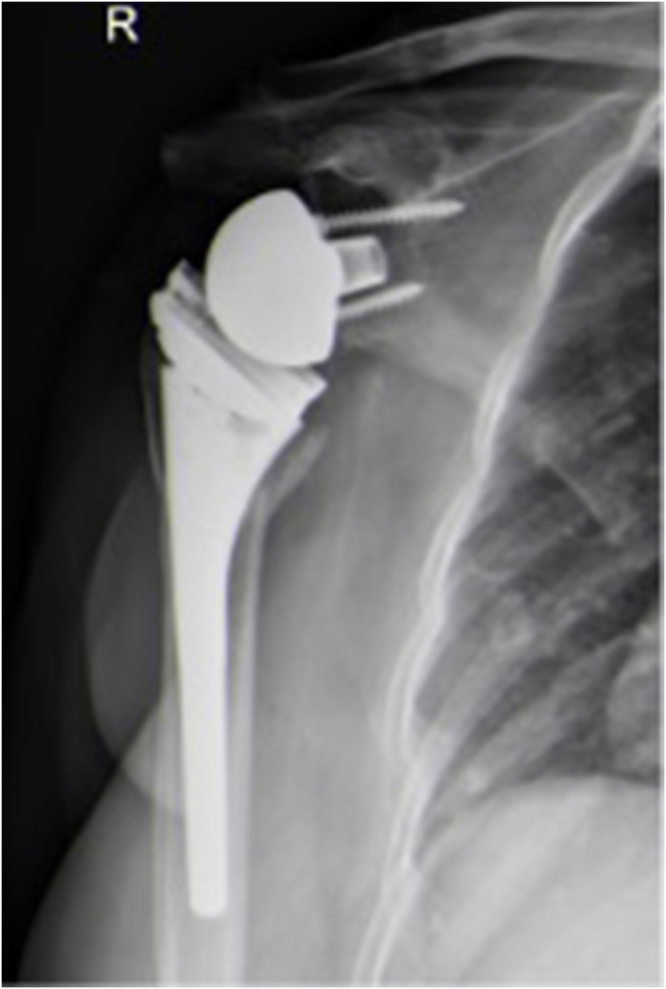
Postoperative X-ray of primary reverse shoulder arthroplasty.
